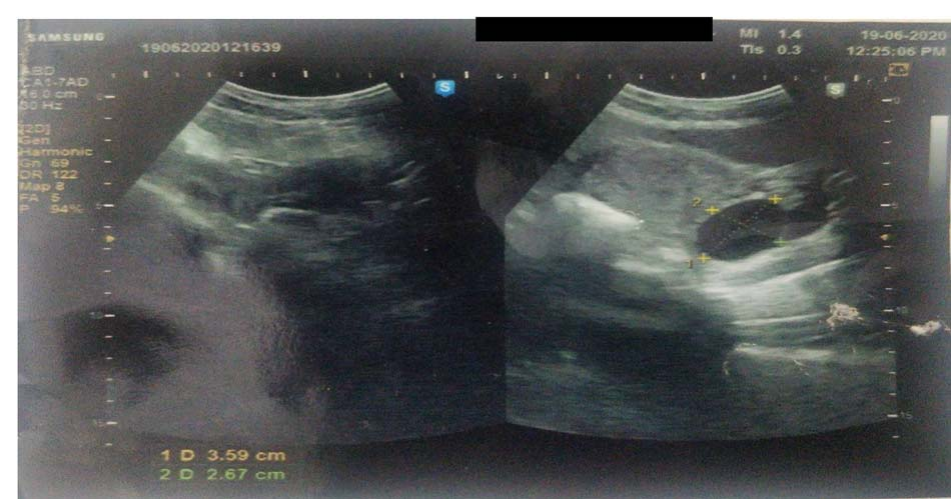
Ultrasound showing a well-defined tubular cystic structure with clear contents in the right inguinal canal up to labia majora measuring 5 cm × 3.5 cm × 5 cm with a volume of 50–60 ml.
